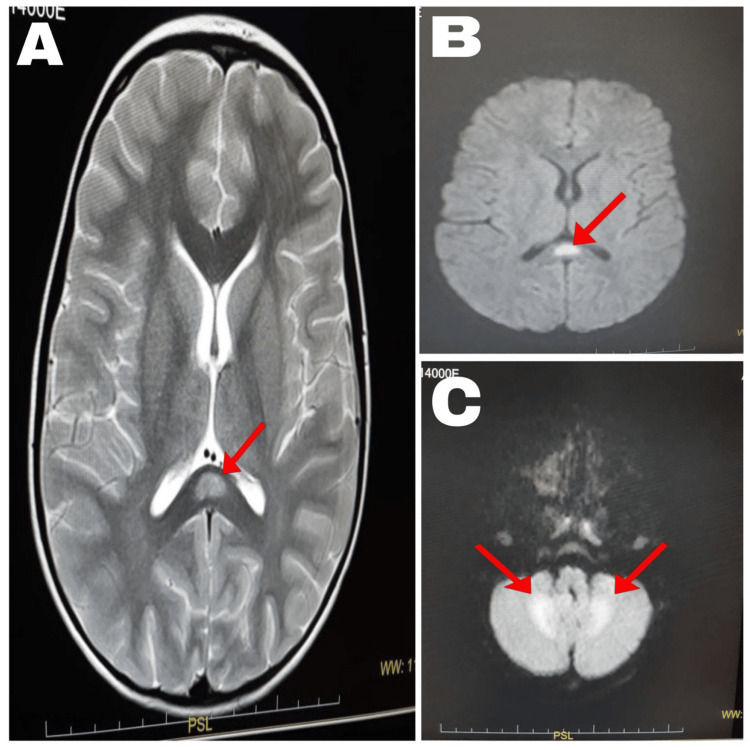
Initial brain magnetic resonance images showing splenial and bilateral cerebellar lesions. (A) Axial T2-weighted image showing a focal hyperintense lesion in the splenium of the corpus callosum (red arrow). (B) Axial fluid-attenuated inversion recovery image confirming the splenial lesion (red arrow). (C) Axial diffusion-weighted image showing bilateral cerebellar lesions involving the dentate nuclei (red arrows).
